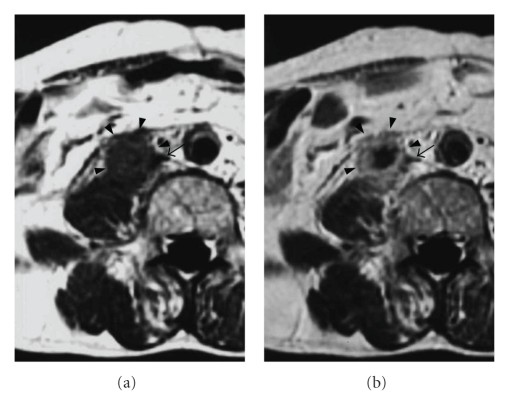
(a) T1-weighted magnetic resonance images demonstrated a retroperitoneal mass (arrowheads) with compression of the inferior vena (arrow). (b) Contrast-enhanced T1-weighted image showed the mass in a ring-like fashion.
